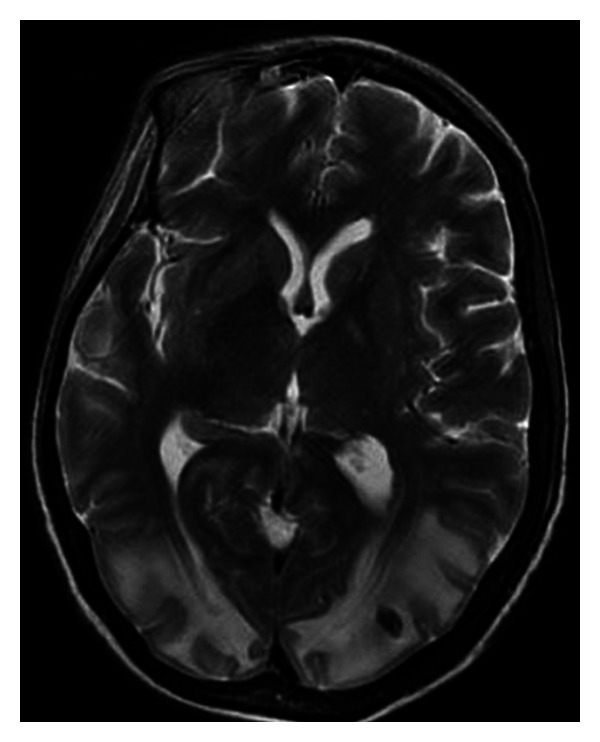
Axial T2-weighted MRI image without contrast demonstrating hyperintensity in the occipital and parietal regions bilaterally, with small foci hemorrhage in the parieto-occipital region.
